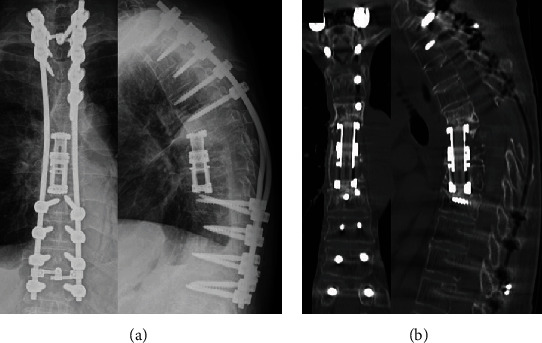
Imaging findings at the final follow-up. (a) X-ray. T1-12 posterior fusion and T6-7-8 corpectomy and anterior reconstruction with an expandable case were performed. (b) CT sagittal and coronal reconstruction. Complete bony fusion was observed inside the cage.
